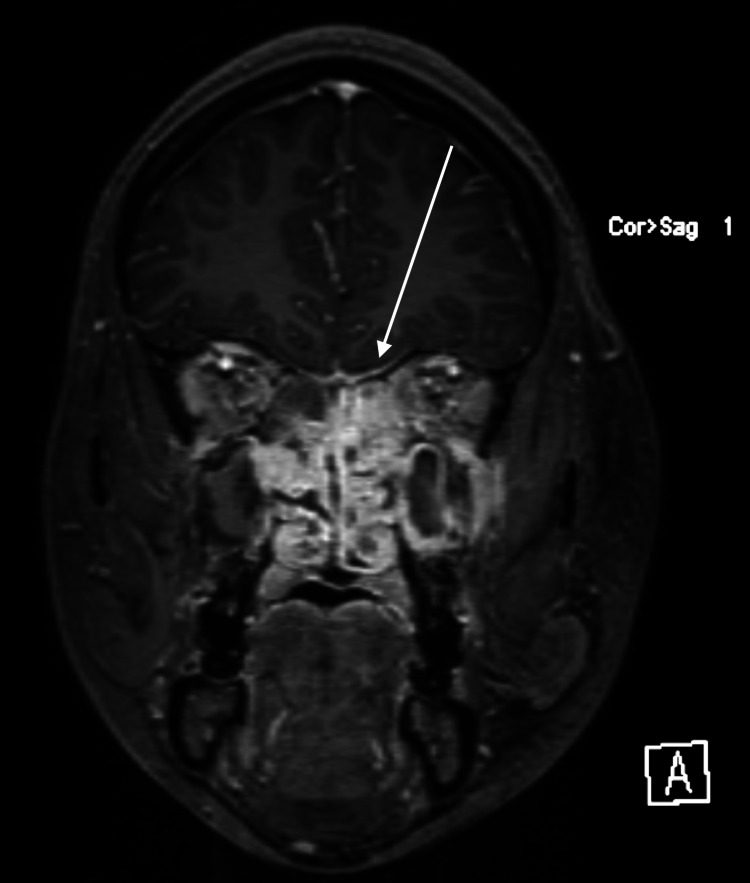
MRI with contrast of the head and maxillofacial area demonstrating inflammation of the left maxillary and sphenoid sinus cavities as well as orbital involvement. Additional linear dural enhancement along the anterior cranial fossa floor without brain parenchyma involvement demonstrates early epidural involvement (white arrow).
